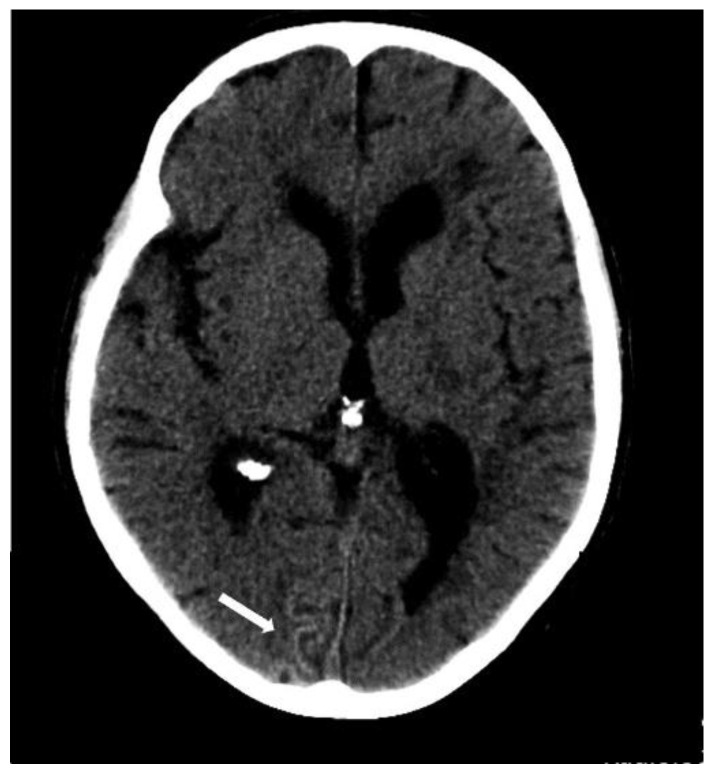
Gyriform calcification of right occipital cortex (arrow) on non-enhanced CT (image courtesy I. Rasing, Leiden/The Netherlands).
